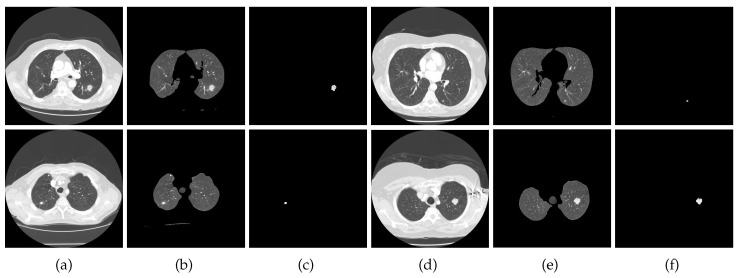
Nodule detection results: (a,d) input CT slice, (b,e) segmented lungs, (c,f) detected nodule.
